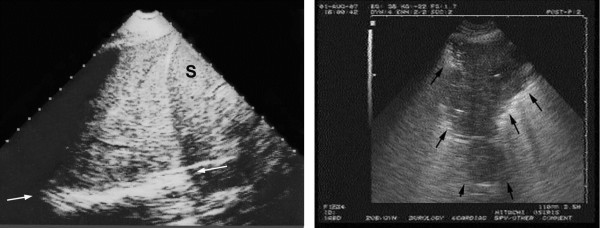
Lung consolidation. Two signs of lung consolidation. Left: a massive consolidation (probe at the PLAPS-point) invades the whole left lower lobe. No aerated lung tissue is present, and no fractal sign can be generated. The deep border is at the mediastinal line (arrows). The pattern is tissue-like, similar to the spleen (S). The thickness of this image is roughly 10 cm, a value incompatible with a pleural effusion. Image acquired using an ADR-4000 and a sectorial probe (1982 mobile technology) Right: a middle lobe consolidation, which does not invade the whole lobe. This generates a shredded, fractal boundary between the consolidation and the underlying aerated lung (arrows): the quite specific shred (or fractal) sign. Such an anterior consolidation generates the C-profile in the BLUE-protocol. Compare with the regular lung line of Figure 3. Note the blurred letters due to multiple transfers of this image. Quantitative data: a reasonable thickness at the right image is 5.5 cm, giving an index of 5.5 corresponding to a 165-mL consolidation, roughly. In the left image, the 10-cm depth would correspond to a volume of roughly 1 L. Adapted from “Whole body ultrasonography in the critically ill” (2010 Ed, Chapter 16), with kind permission of Springer Science.
